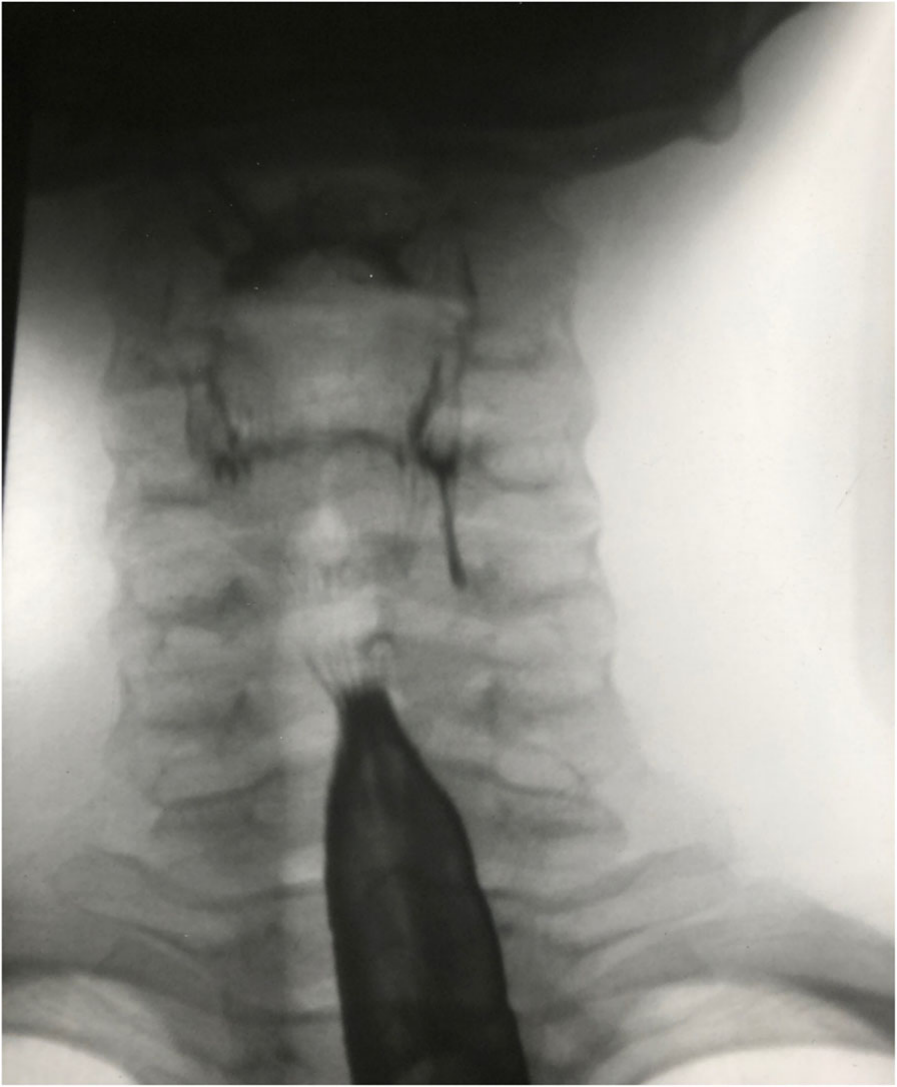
Barium swallow with rapid sequencing images showing a 4 cm. blind-ended sinus from the apex of the left piriform fossa extending inferiorly in relation to the deep aspect of the lobe of the thyroid. Additional imaging was undertaken with ultrasound
